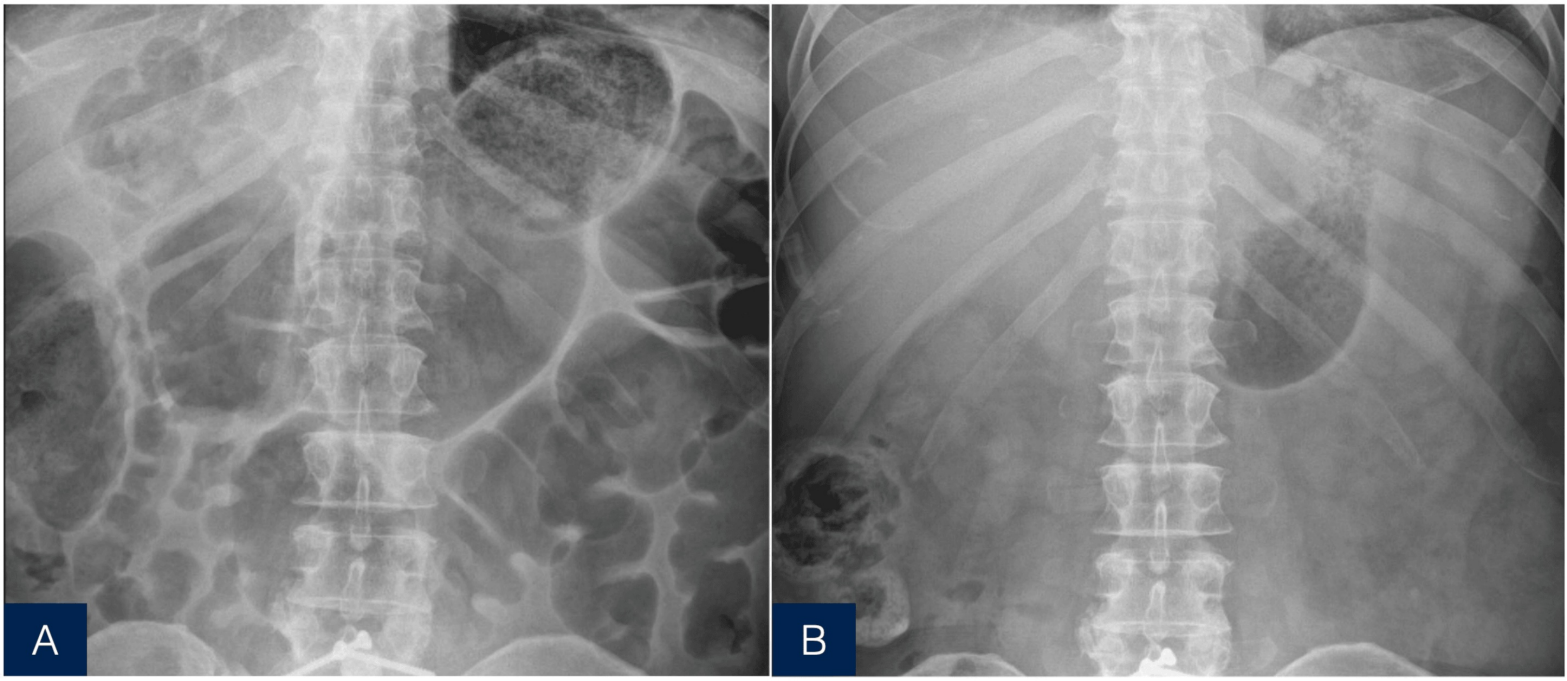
Plain film abdominal radiographs before and after treatment The plain film abdominal radiograph before treatment (A) showed markedly dilated intestines and gastric distention before escalation to methylprednisolone. After treatment (B), there was an improvement in dilatation and distension. This was two weeks after initiation of high-dose immunosuppression.
